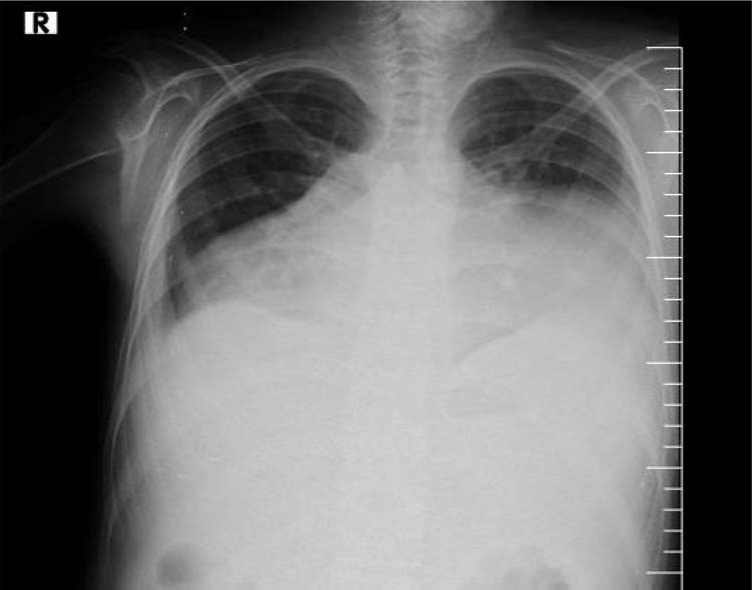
Chest X-rays show an enlarged cardiothoracic ratio, a lack of cardiac silhouette and pleural effusion.
